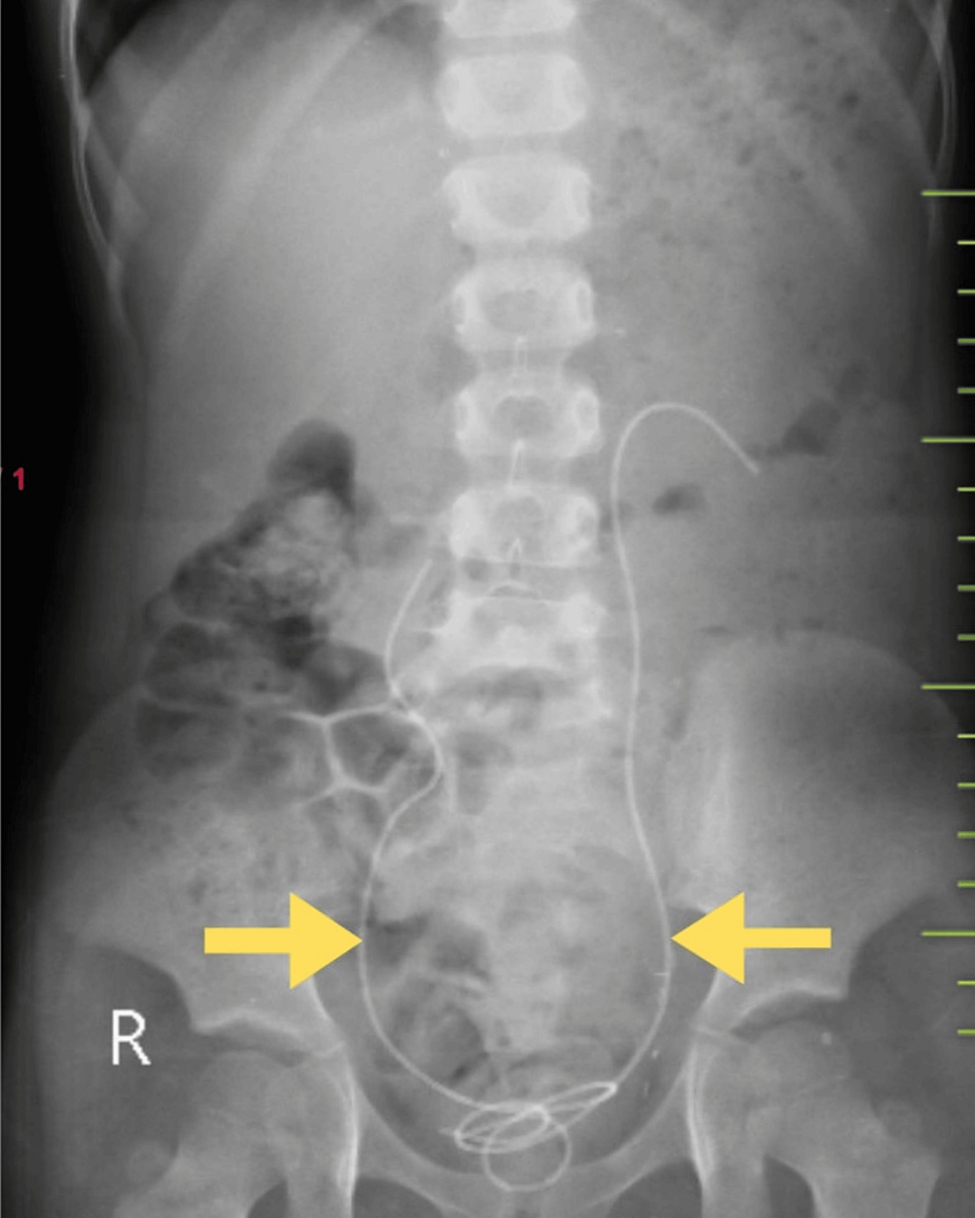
Postoperative KUB X-ray showing bilateral DJ stents KUB: kidney, ureter, and bladder; DJ: double J
